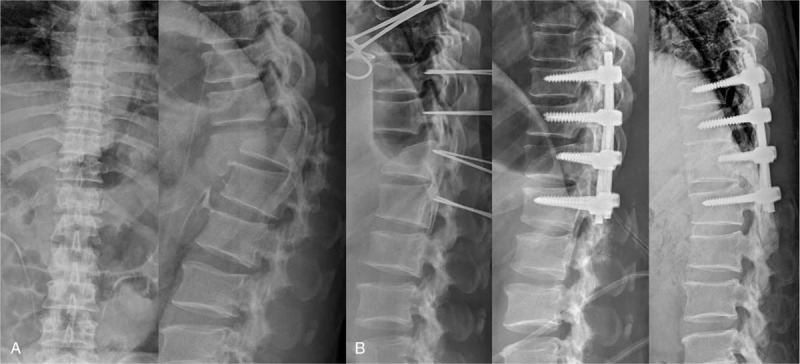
A. Anteroposterior and lateral radiograph of a patient presenting after injury with fracture-dislocation injury at T11/T12. B. Intraoperative K-wire X-ray and proper alignment of vertebrae immediately after the operation and at the 4-year follow-up.
